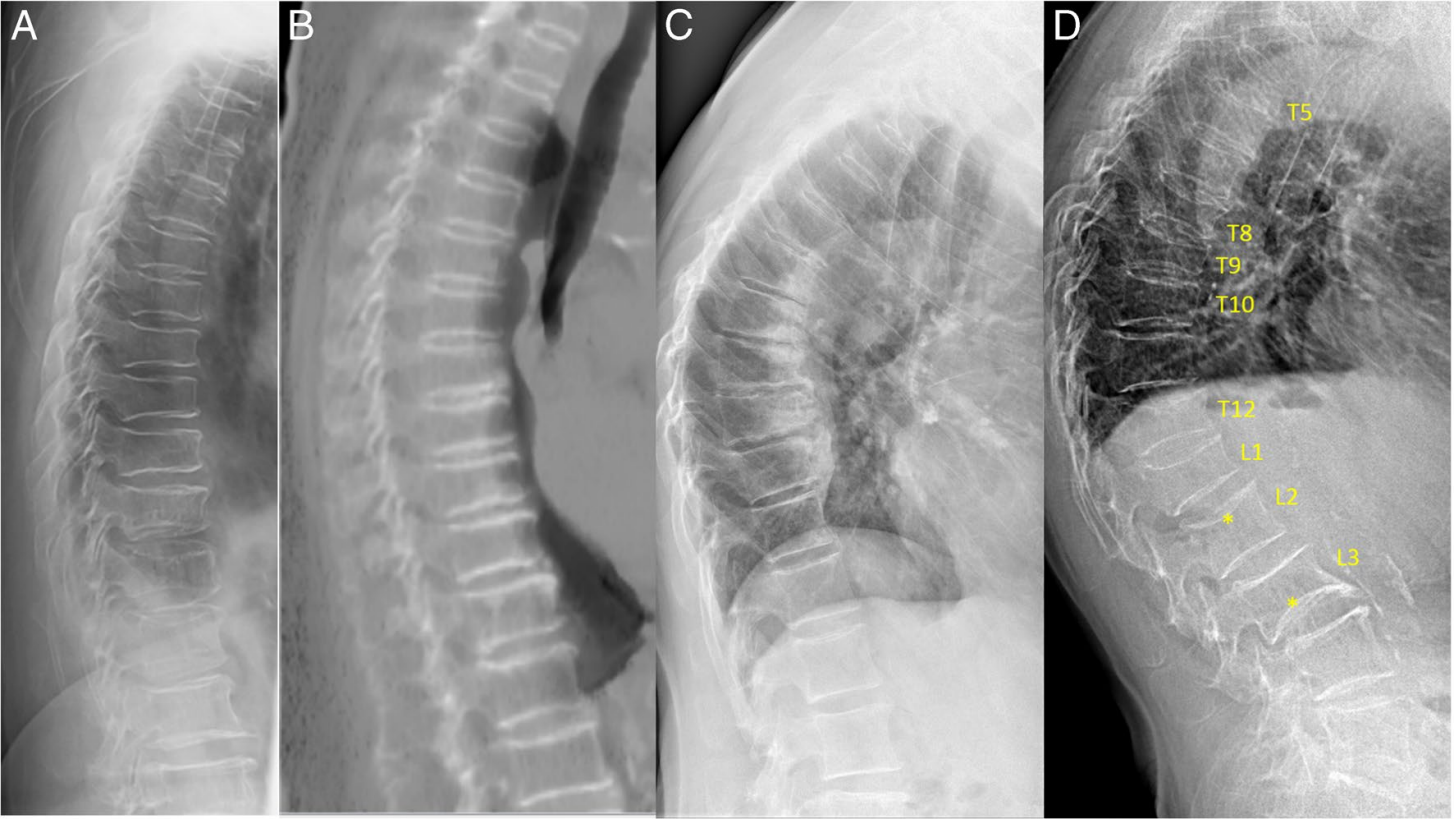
Differentiation of multiple SVa, multiple osteoarthritic (OA) wedging, and multiple OLVFs. A Lateral radiograph in a female shows multiple SVa. B Sagittal CT reconstruction on another female patient shows multiple SVa. C Lateral radiograph demonstrating multiple-level OA wedging in a female patient. D Lateral radiograph in a female patient demonstrates multiple OLVFs. In A and B, the multiple SVa show much less variation in shape and severity, and there is no wedging or apparent endplate depression. In B, the increased density of the involved endplates suggests regenerative inflammatory changes. While the many SVa in (A) and (B) are already quite severe, there is no apparent fracture. OA wedging in (C) involves multiple vertebrae at the mid-thoracic spine, and these vertebrae show a similar degree of wedging, with osteophytes. In D, OLVFs vary greatly in shape and severity, and most of them show endplate depression. There is a superior endplate fracture (depression) in L2 (asterisk); L3 demonstrates an expansive inferior endplate (anomaly) (asterisk). SVa, acquired short vertebrae; OLVF, multiple osteoporotic-like vertebral fracture. Reproduced with permission from Wáng. Quant Imaging Med Surg. 2023;13:1264–1285
